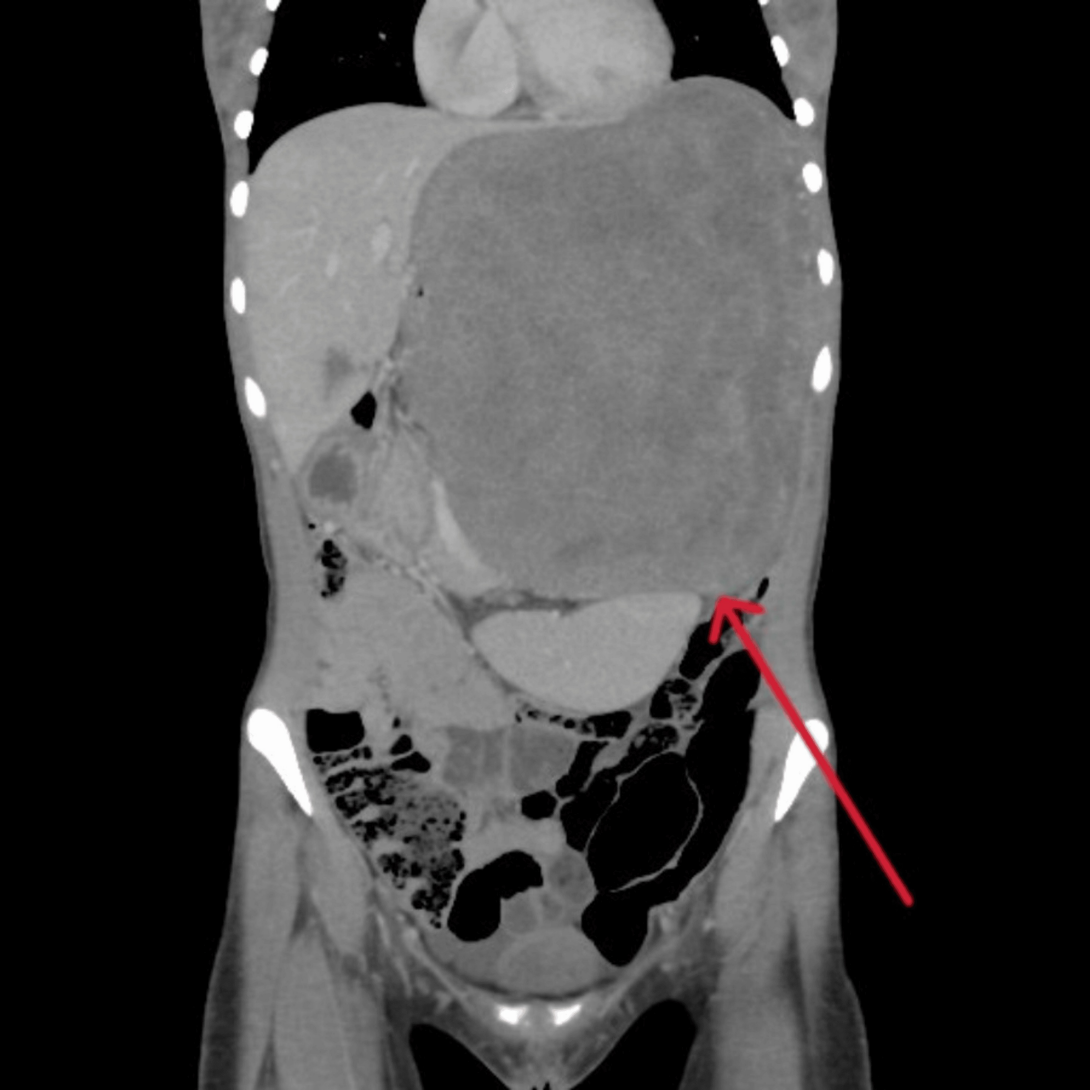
Coronal section of the abdomen and pelvis on CT The coronal cross-sectional CT image of this patient reveals a massive left retroperitoneal soft tissue lesion measuring 17 × 12 × 21 cm.
